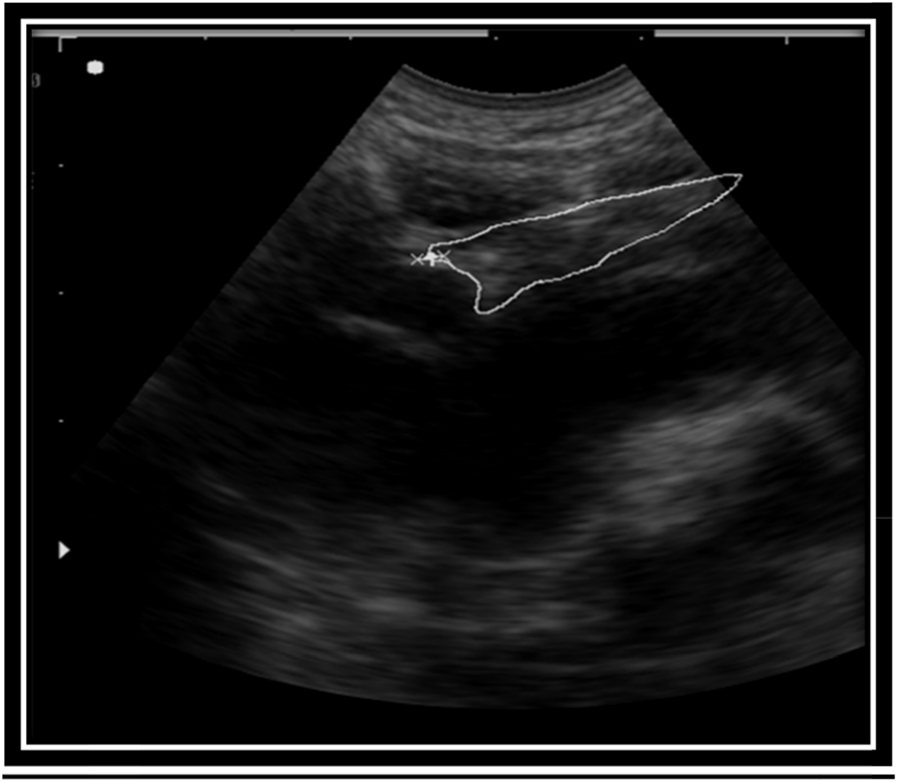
Ultrasound scan of a child with SAM
